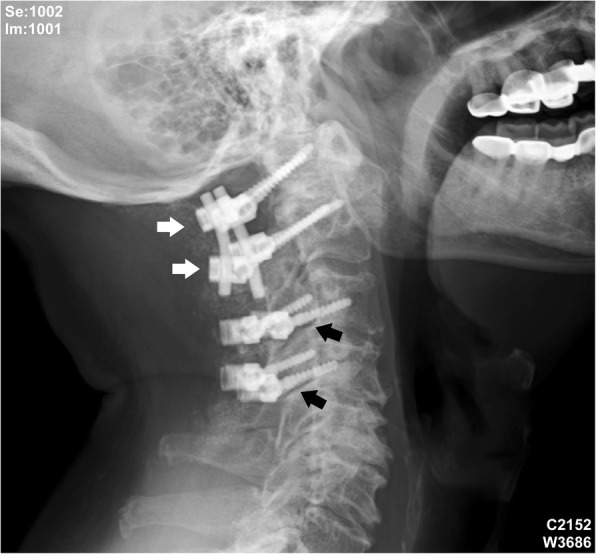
CT image after C1-C2 instrumentation. The white arrows indicated the newly operated C1-C2 instruments, and the black arrow indicated the original occipito-C3-C4 fixation instruments
